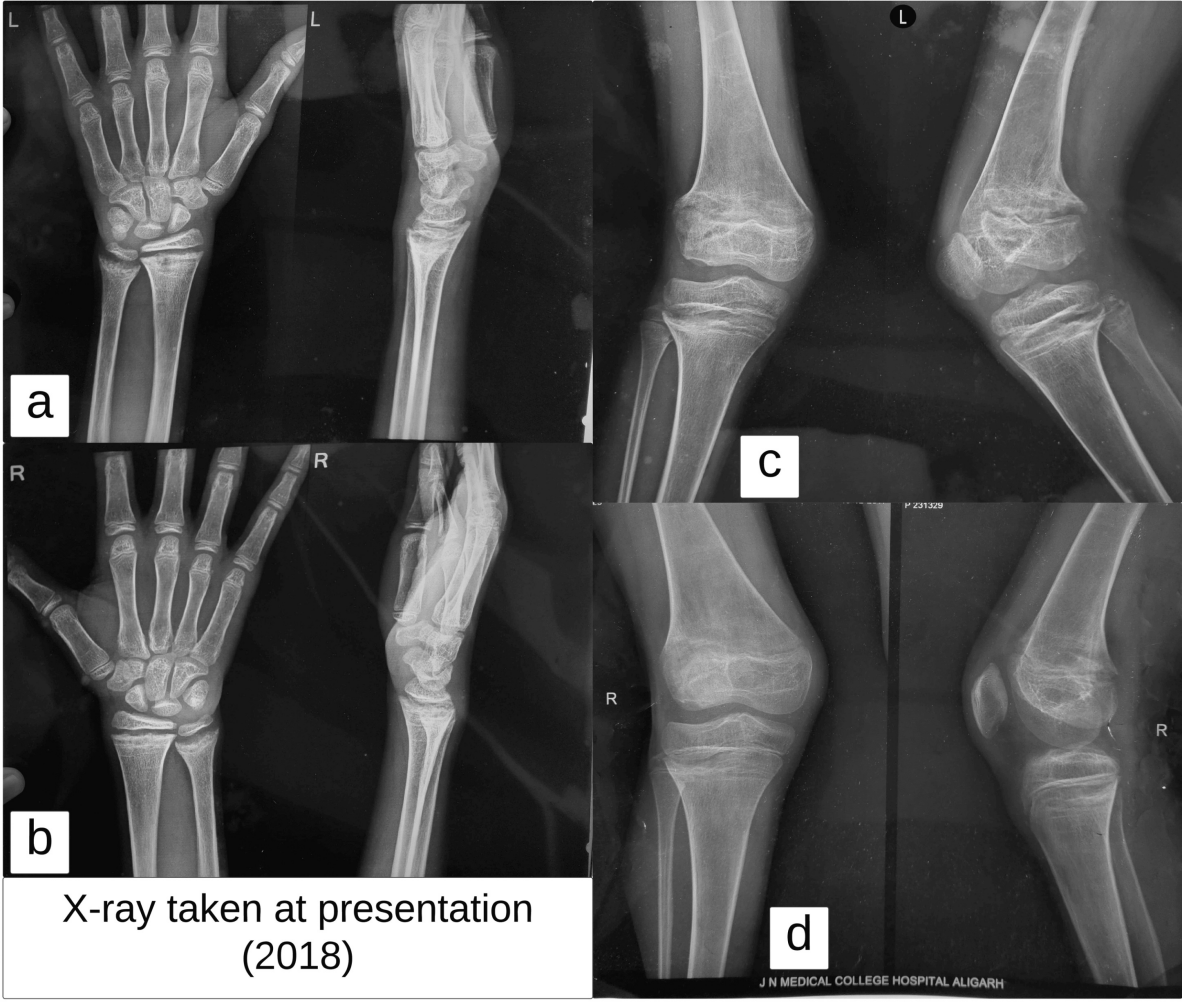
(a) and (b): X-ray bilateral hand with wrist showing widening, fraying, and cupping of the metaphyseal ends of the distal radius and ulna; prominent widening of the growth plates and generalized osteopenia; (c) and (d): X-ray bilateral knee showing bilateral genu valgum deformity with widening and cupping of the metaphyseal ends of the femur and tibia, widened and irregular growth plates, and reduction in the cortical thickness of the long bones
